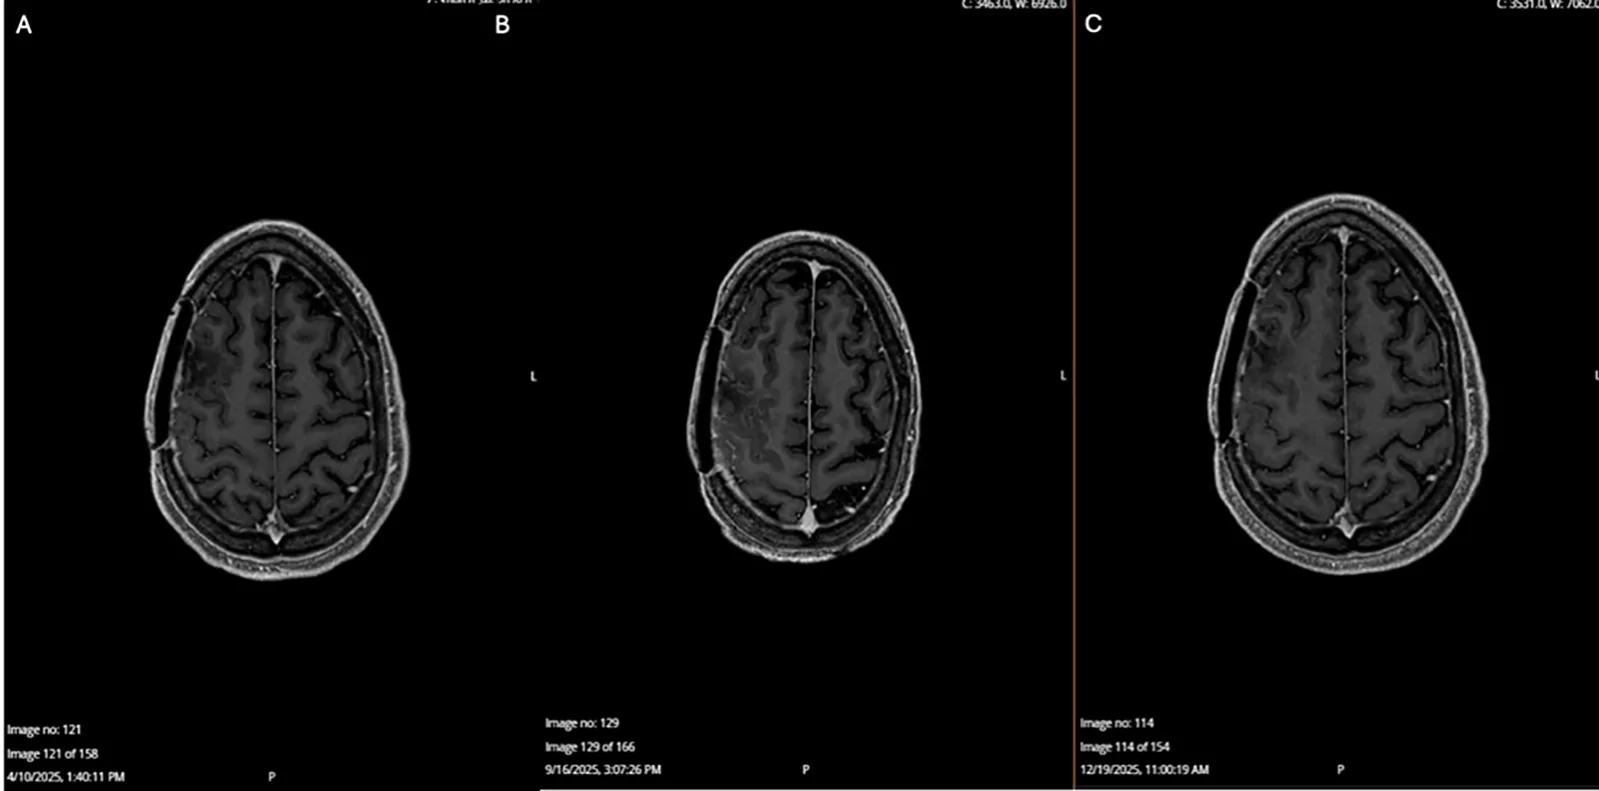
(A–C) Axial T1 MRI series with and without gadolinium contrast of the temporal progression of SMART syndrome changes from his baseline MRI with and without contrast before symptom onset that shows findings of right frontal craniectomy and cranioplasty (A), the peak sulcal enhancement upon presentation on the right cortical/leptomeningeal FLAIR hyperintensity with associated enhancement beneath the cranioplasty peripherally (B), and the rapid improvement with interval resolution of the cortical mantle diffusion restriction and pathologic superficial enhancement subjacent to the cranioplasty site after high dose steroids when re-imaged three months later (C).
